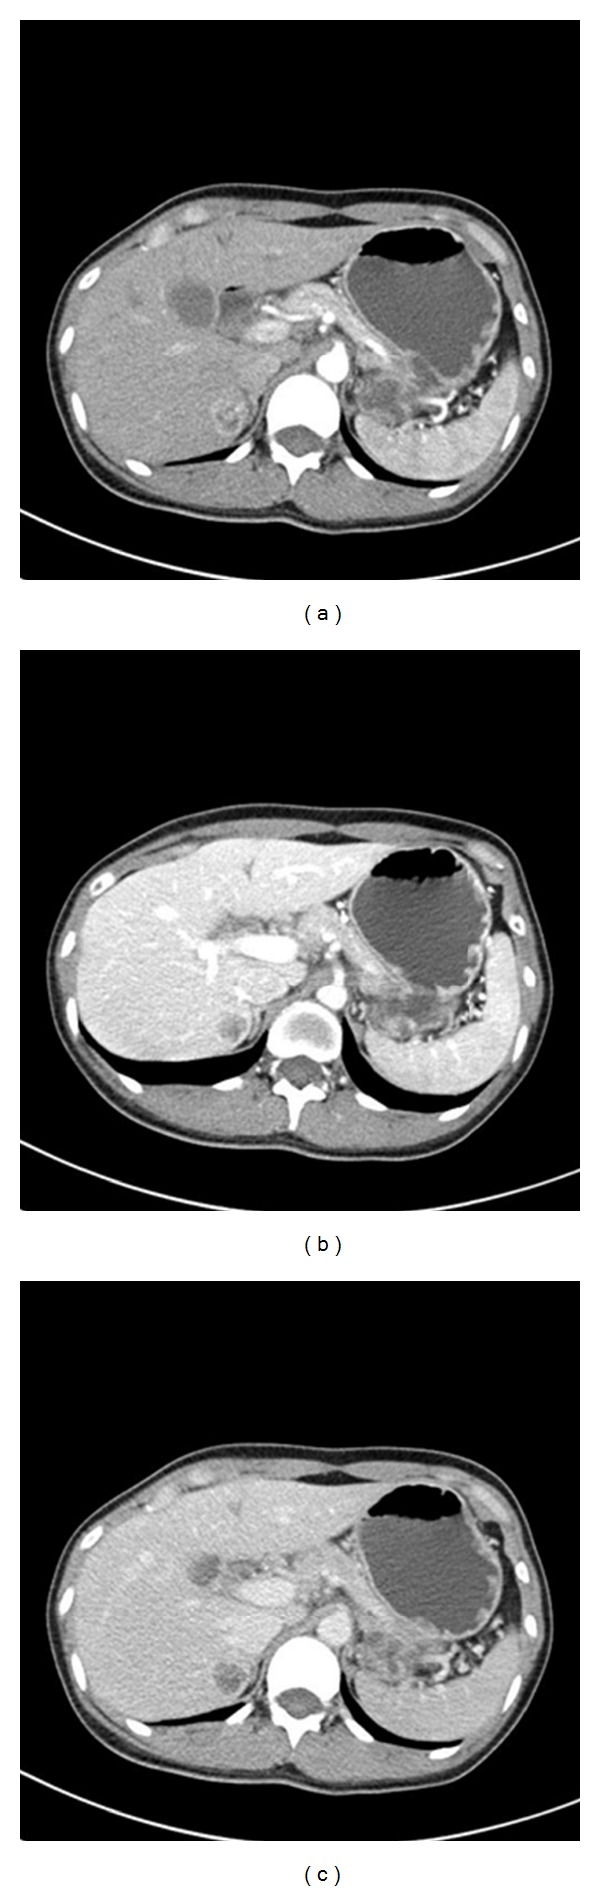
CT findings of the liver mass. Contrast-enhanced CT revealed a heterogeneous hypervascular mass in the arterial phase (a) and washing-out of the medium in the portal (b) and delayed phases (c).
